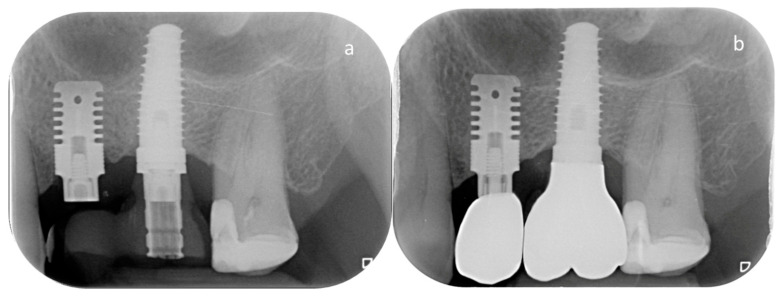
Periapical radiographs taken at baseline (T0) (a) and after 7 years (b) to evaluate marginal bone changes.
